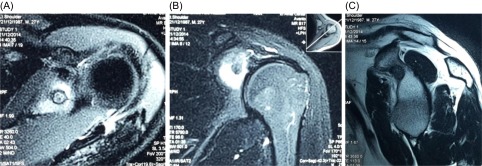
(A and B) T2-weighted MRI images showing high signal intensity within the glenoid around the lesion with low signal, (C) T1-weighted sagittal image reveals a low signal lesion at the base of the corocoid.
